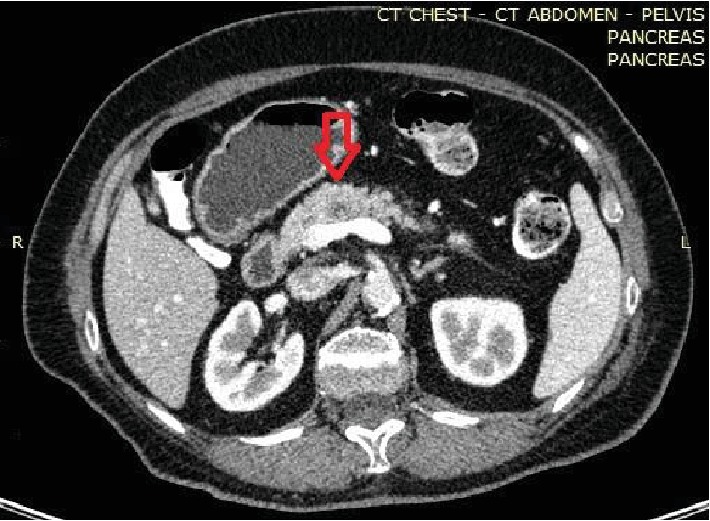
Computed tomography (CT image) demonstrating a hypodense pancreatic mass (red arrow) anterior to the superior mesenteric vein and splenic vein confluence.
